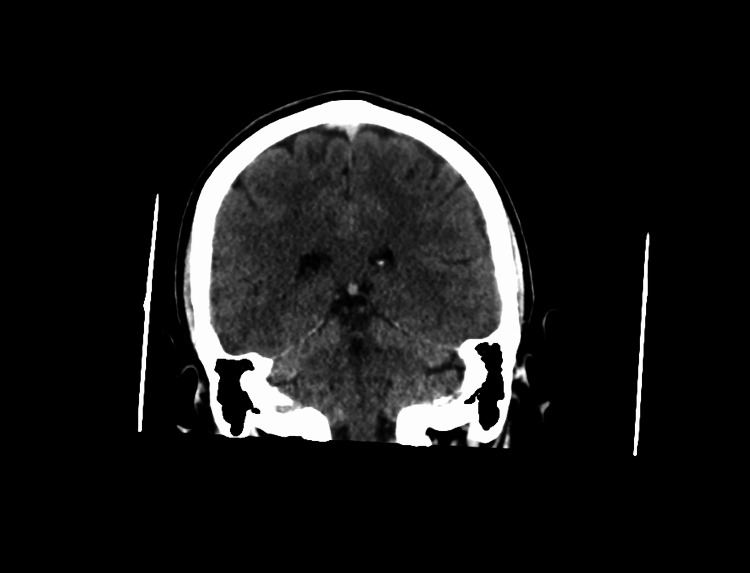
CT head without contrast (coronal view)
